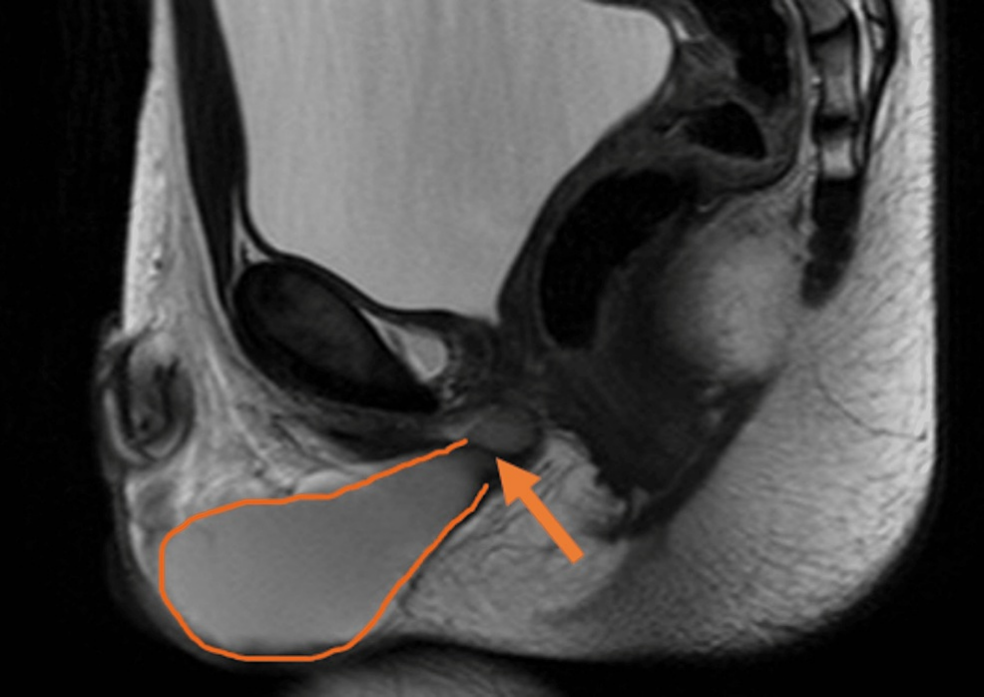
Pelvic MRI. The boundaries of the cyst have been outlined in orange, and the stalk connecting it to the base of the prostate has been marked with an orange arrow. MRI, magnetic resonance imaging
